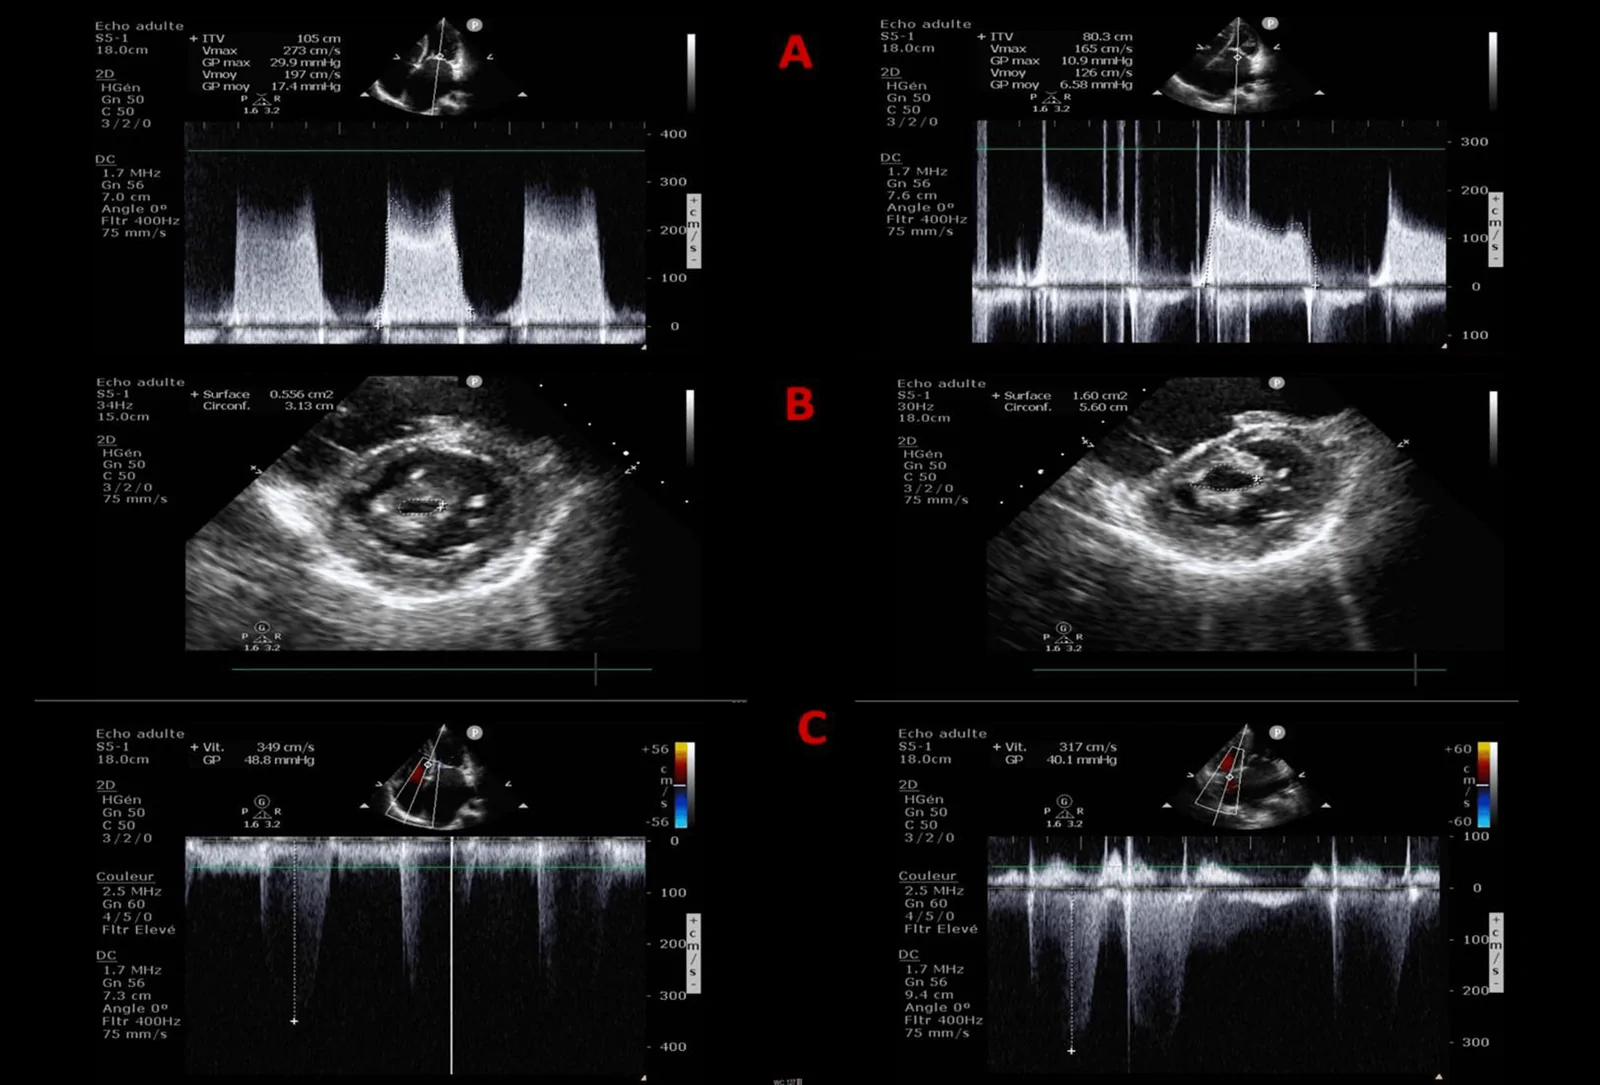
Transthoracic echocardiography before and after percutaneous mitral commissurotomy Left panels: baseline study. Right panels: study repeated immediately after the procedure. (A) Continuous-wave Doppler across the mitral valve, showing a mean transmitral gradient of 17.4 mm Hg at baseline and 6.6 mm Hg after commissurotomy. (B) Parasternal short-axis planimetry of the mitral orifice, showing a valve area of 0.55 cm² at baseline and 1.60 cm² after commissurotomy. (C) Continuous-wave Doppler of the tricuspid regurgitation jet, showing a peak gradient of 48.8 mm Hg at baseline and 40.1 mm Hg after commissurotomy.
